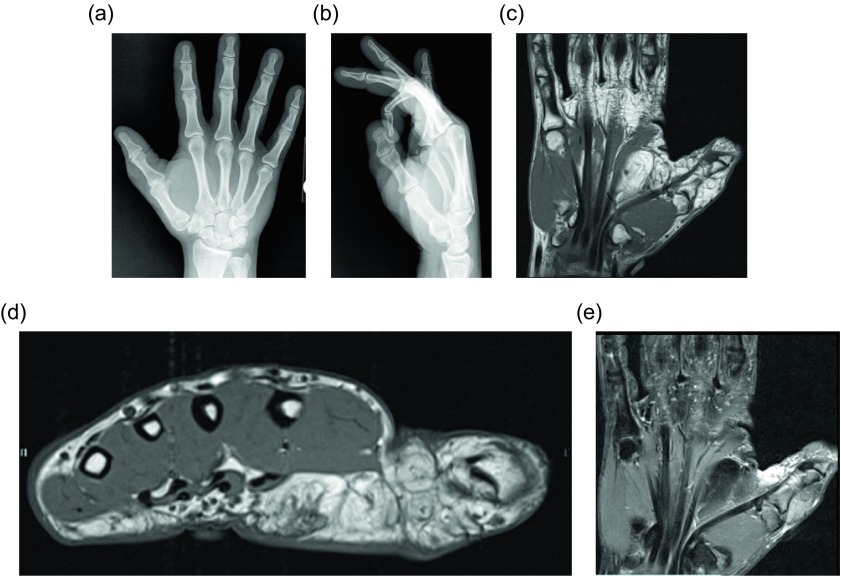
Fibrolipomatous hamartoma: 34-year-old male with slowly enlarging soft tissue mass of the right thumb. Frontal (a) and lateral (b) radiographs of the right hand shows soft tissue fullness of the right first digit and thenar eminence. Coronal (c) and axial (d) T1W and coronal (e) T2W FS (fat-suppressed) images demonstrate thickening of the digital nerve extending to the first digit with lipomatous hypertrophy of the first digit soft tissues.
